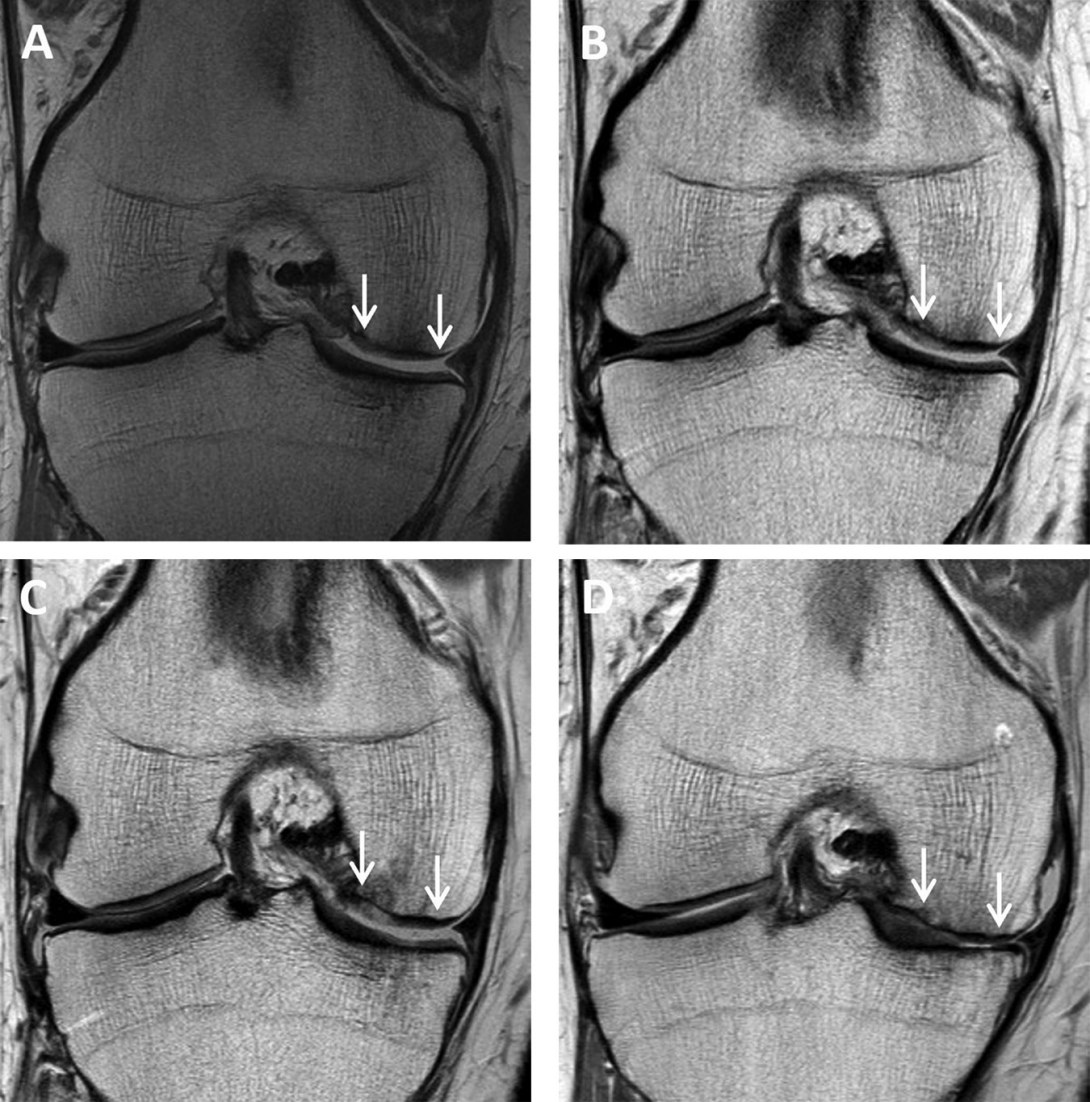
Proton density fast spin-echo magnetic resonance images of a matrix-induced autologous chondrocyte implantation (MACI) graft (between white arrows) on the medial femoral condyle at (A) 3 months, (B) 1 year, (C) 2 years, and (D) 5 years, with 5-year imaging reflecting a failed graft and this patient subsequently undergoing knee replacement surgery at 8 years after the primary MACI surgery.
